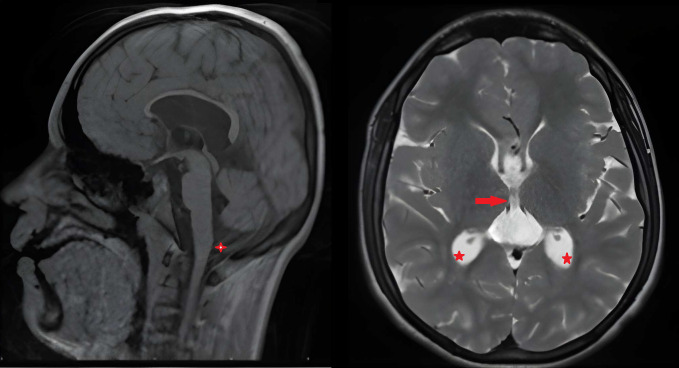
Sagittal T1-weighted and axial T2-weighted brain MRI of Case 1 demonstrating enlargement of the cisterna magna/cisterna magna permagna (indicated by a cross +), dilation of the third ventricle with communication with the cavum vergae (indicated by an arrow →), and colpocephaly (indicated by a star ☆).
